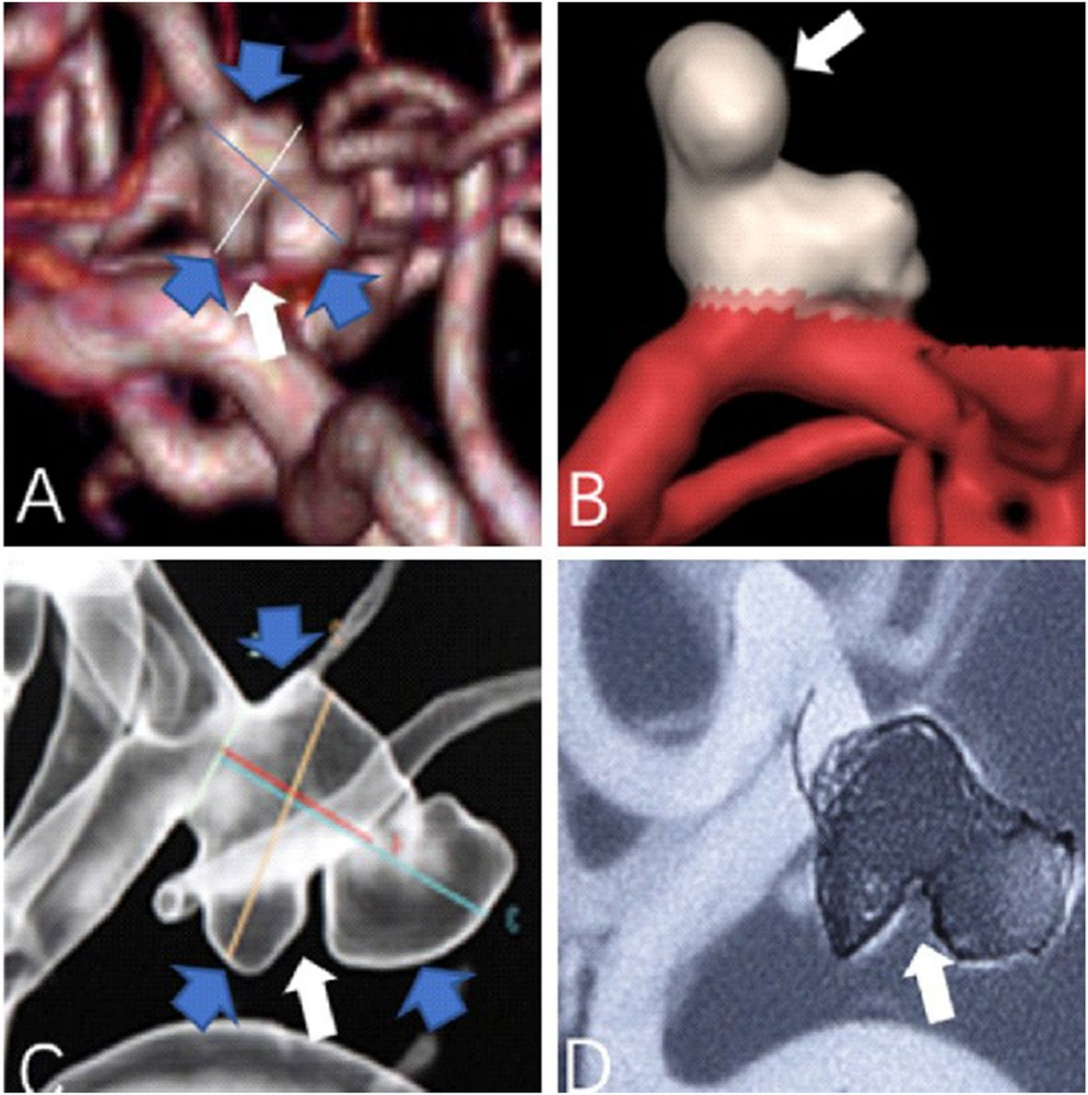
A-B CTA showed a left posterior communicating artery aneurysm (PcomA) (10.2 mm x 7.8 mm), and an obvious pulsation was observed by 4D-CTA (as shown by the white arrow). Both observers reported a large aneurysm, having more than two lobes (as shown by the blue arrow). a narrow neck, and clear visualization of parent and branch vessels. C-D DSA findings confirmed CTA findings, the patient underwent interventional embolization therapy directly, the therapeutic effect was shown in frame D
